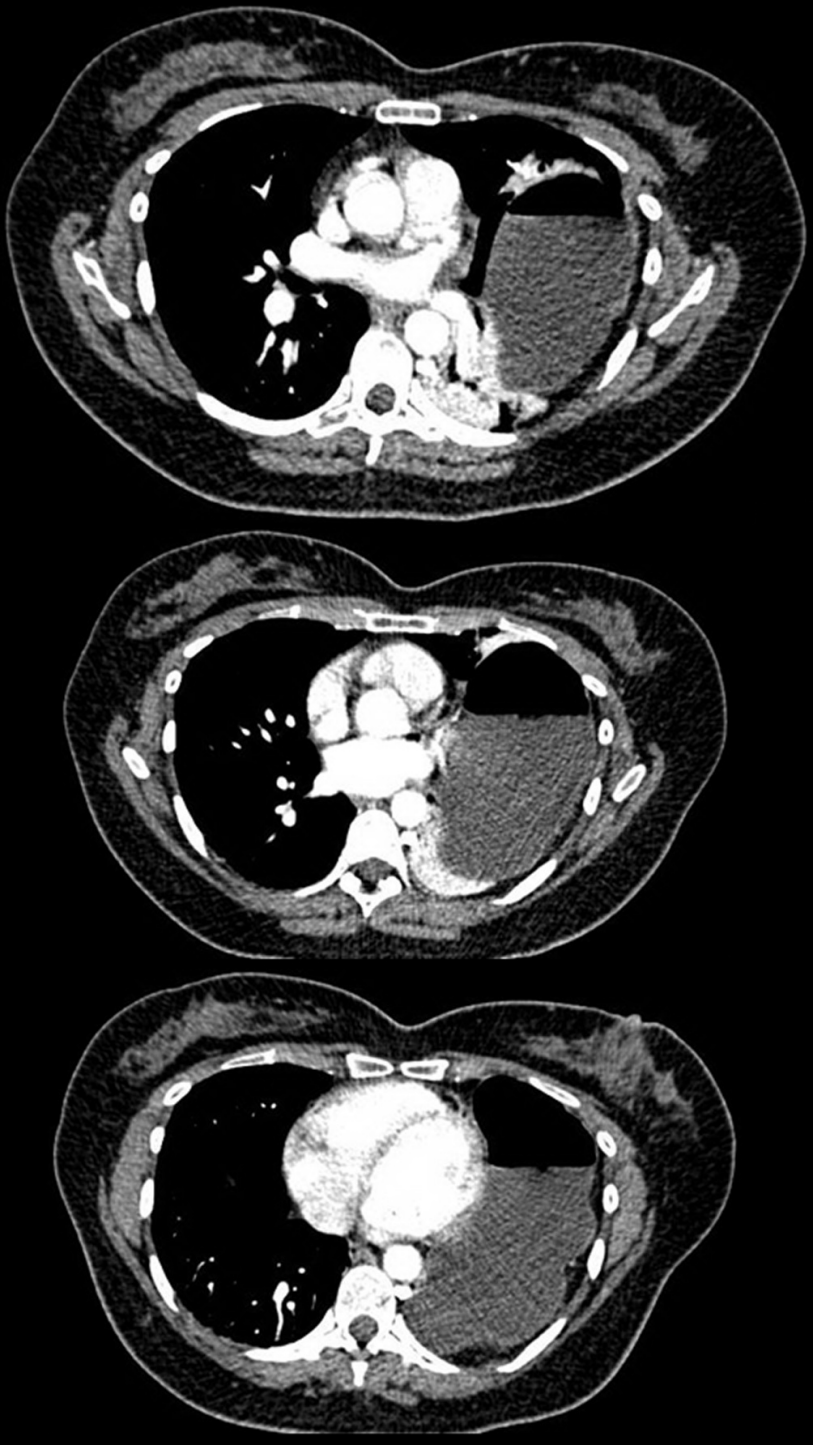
Thoracic computed tomography scans Axial sections of computed tomography scans demonstrate herniation of stomach into the left thoracic cavity.
